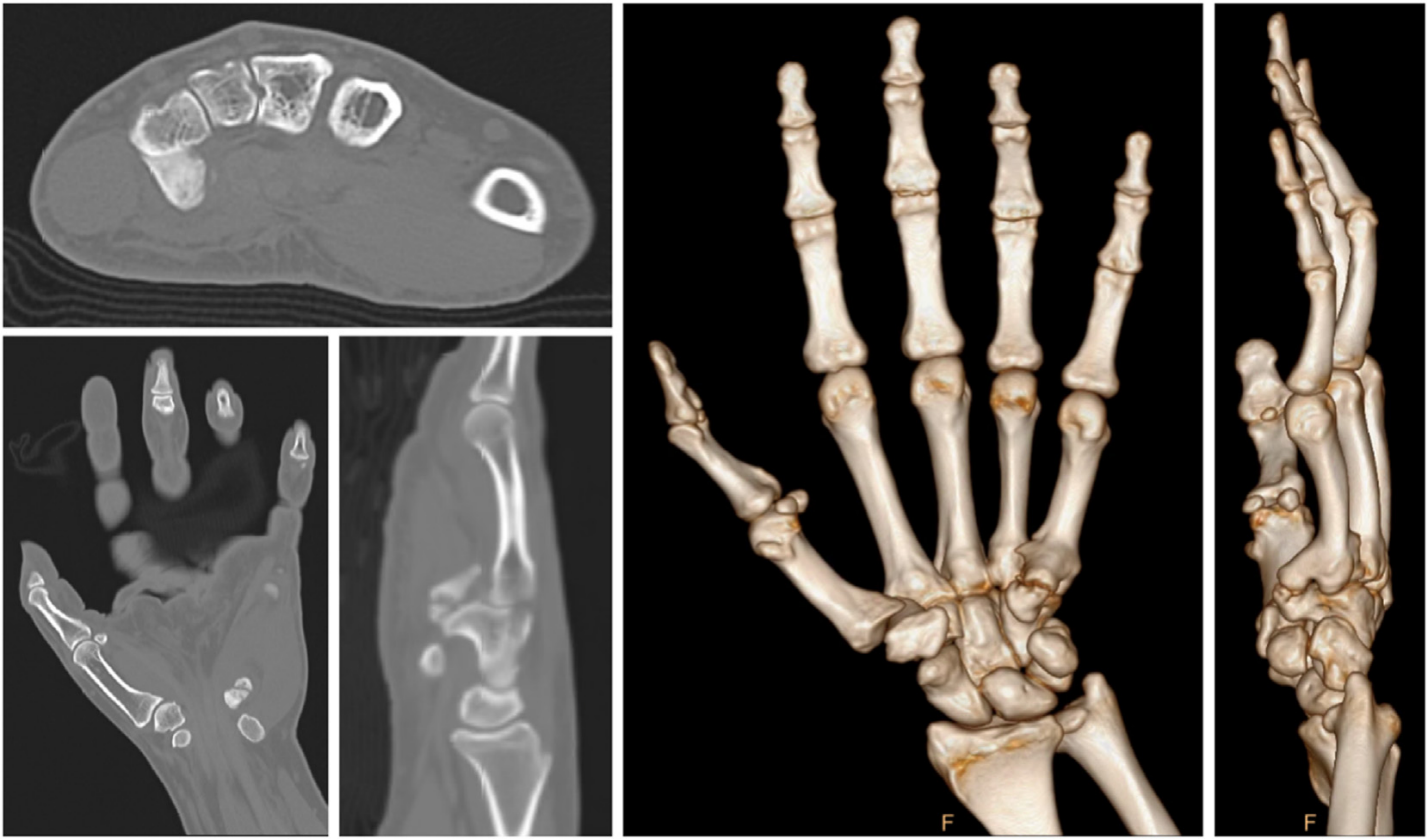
Computed tomography scan and three-dimensional reconstructions of the left hand obtained 6 months after injury demonstrating heterotopic bone formation between the hook of hamate and volar little finger metacarpal base.
